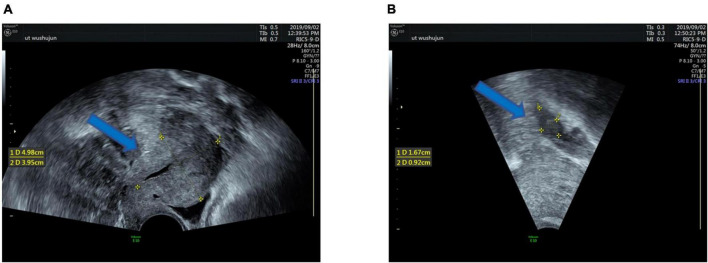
Clinical imaging performance: Ultrasound shows multiple uterine fibroids (A), the maximum 4.98 × 1.95cm size (blue arrow). Ultrasound showing a 1.67 × 0.92 cm right ovarian mass (B) (blue arrow).
